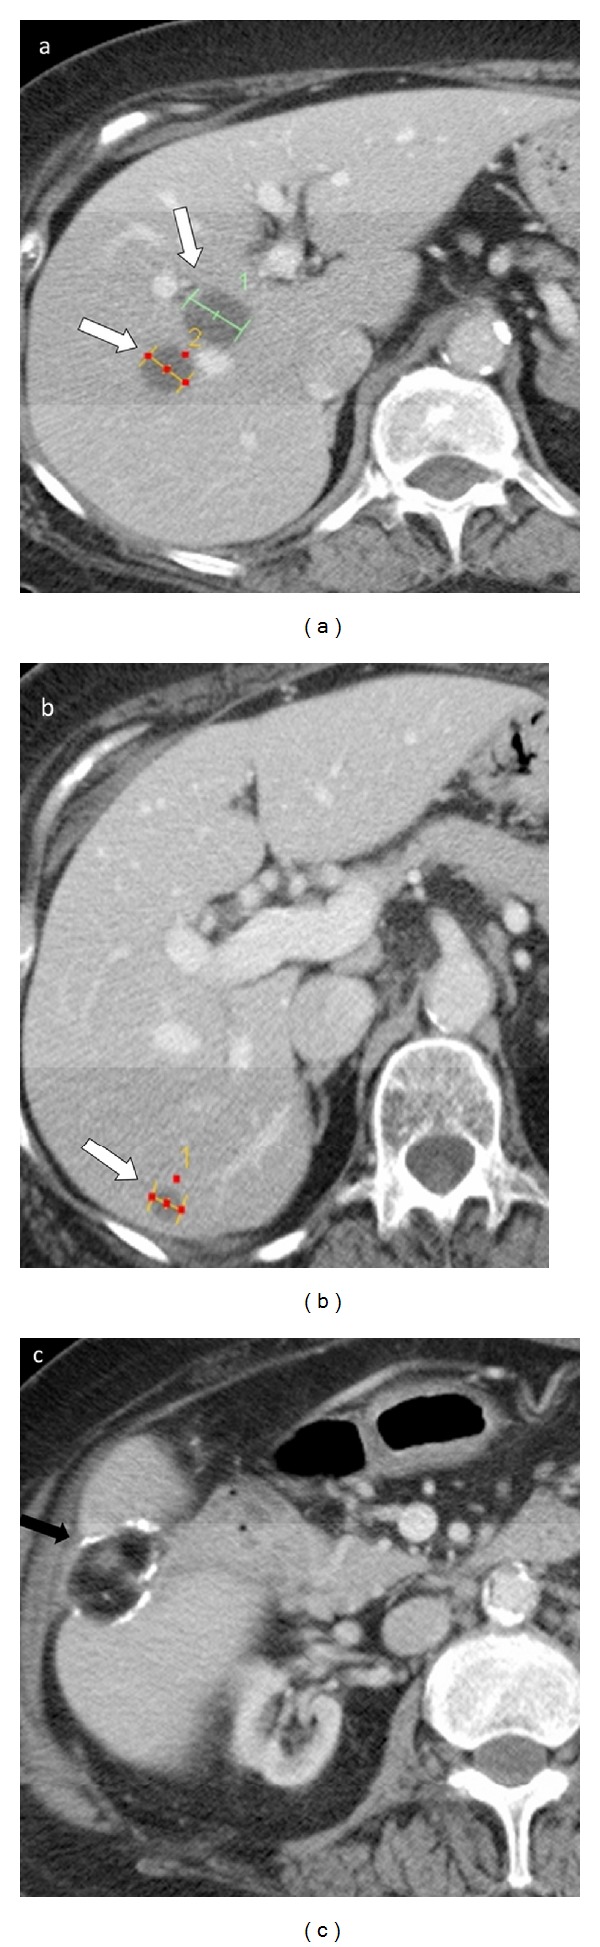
Contrast enhanced CT scan images demonstrate low attenuation right hepatic lobe lesions consistent with known metastatic disease (white arrows, (a) and (b)). Patient is status post partial segmental resection of the right hepatic lobe (black arrow, (c)).
